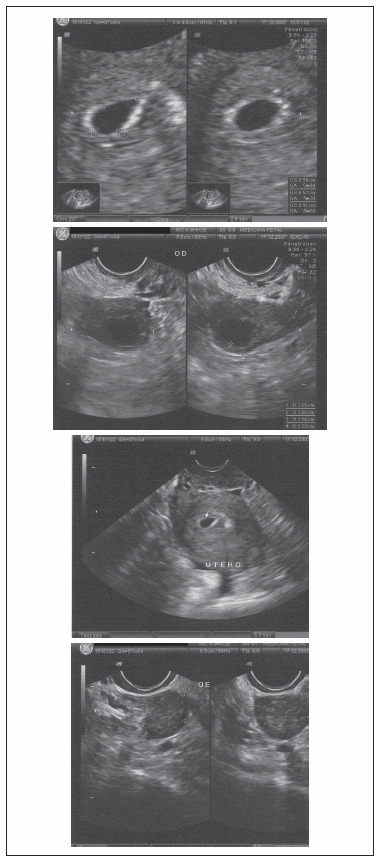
Transvaginal ultrasound of the second intrauterine pregnancy. Left upper quadrant: magnified view of the gestational sac. Right upper quadrant: gestational sac located in the uterus. Left lower quadrant: right ovary with an image suggestive of the corpus luteum. Right lower quadrant: left ovary of normal size.
